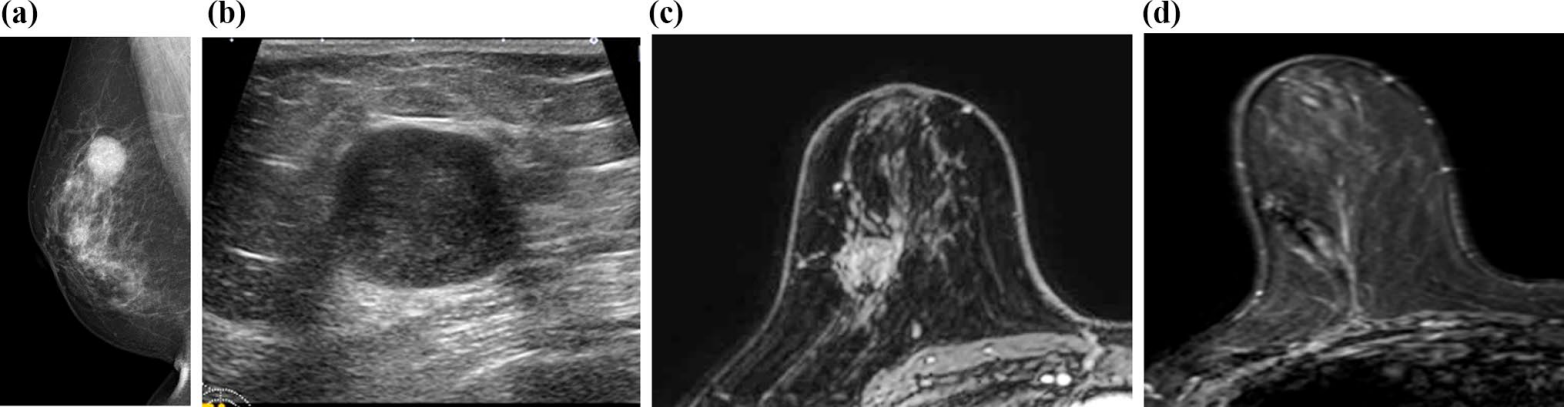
Pre- and post-treatment imaging of a partient in her 70s with TNBC (Case 3). a–c Pretreatment imaging: a Mammography of the right breast, b Ultrasonography of the right breast, c Initial phase of dynamic DCE MRI. Pre-treatment imaging revealed a well-defined, round primary tumor in her right breast (a–c) with two metastatic lymph nodes (not shown). d Post-treatment imaging following neoadjuvant chemotherapy combined with pembrolizumab: Post-treatment scans revealed marked tumor regression, and the patient achieved near-pCR status on DCE MRI (d)
